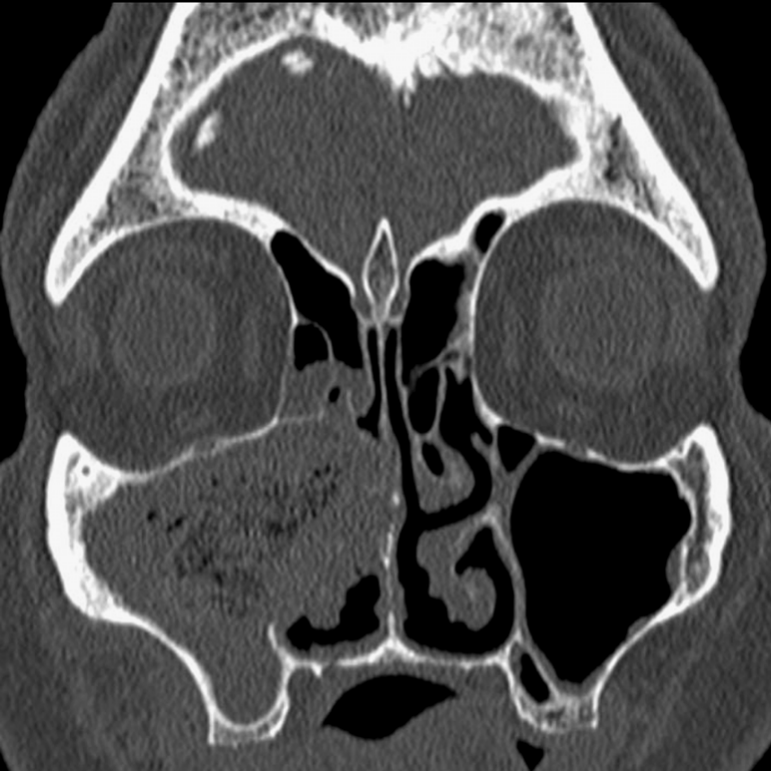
CT scan imaging showing the textiloma located in the maxillary sinus and mimicking a cyst recurrence.
